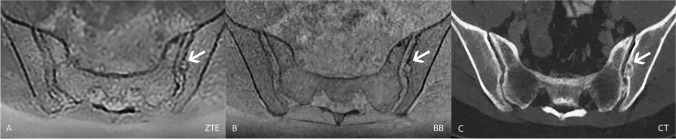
In this 48-year-old female patient referred for a workup of a bowel pathology, erosions were detected on all three transverse images. The erosion (arrow) appeared to be chronic since there was a sclerotic rim visible on the ZTE (image A) and BB (image B) sequence as well as on the CT scan (image C). Abbreviations: ZTE = zero echo time; BB = black bone; CT = computed tomography; SI = sacroiliac joint
